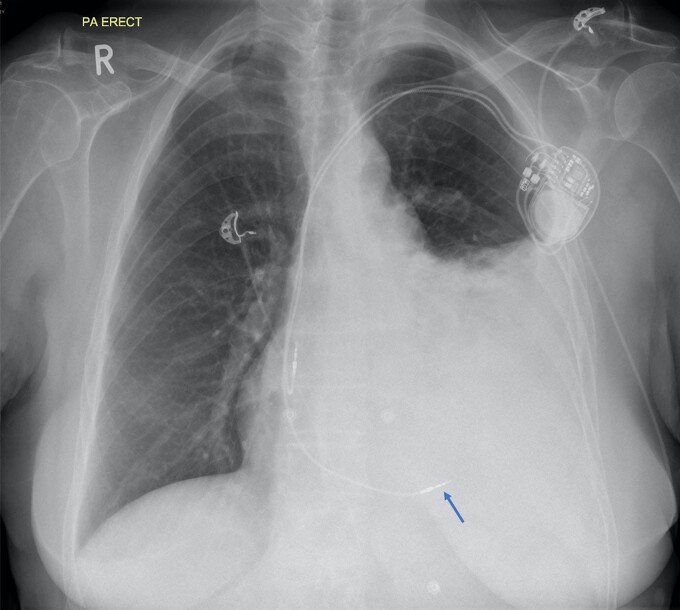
Chest radiograph performed 24 h post procedure demonstrating a new large left pleural effusion and atypical position of right ventricular lead (arrow).
